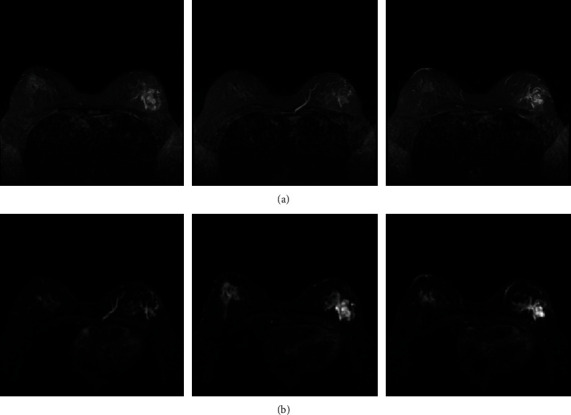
Partially scanned image based on the (a) T2 scan sequence and (b) DWI scan sequence.
